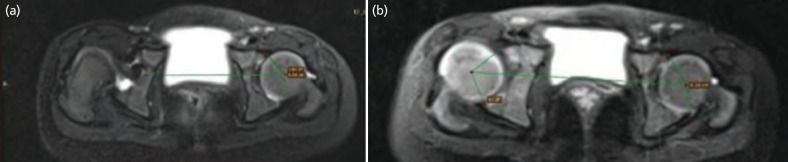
Anterior and Posterior acetabular sector angles (ASA and PSA), (a) T2-weighted fat-saturated image of the hip in a 2-year-old female had right DDH. (b) The same child underwent OR with Salter’s osteotomy for right DDH. The anterior and the posterior sector angles on the right side are 40.1° and 67.8°.
